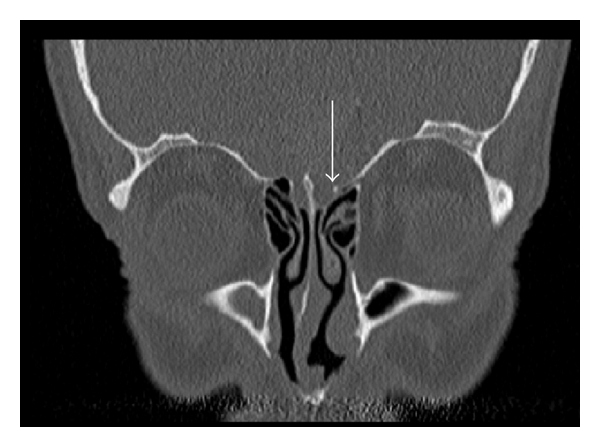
Ethmoid roof is “flattening” or shows a “broken wing” on the left side (arrow). Coronary CT scan of a patient with chronic sinusitis.
